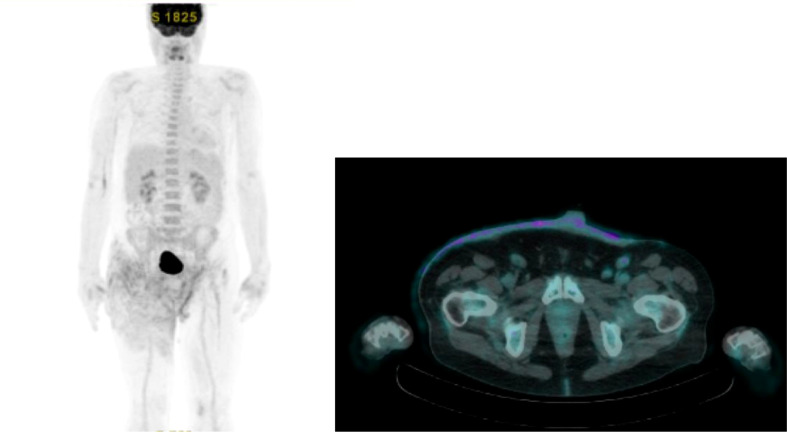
FDG PET/CT scan at baseline of patient’s clinical history.
